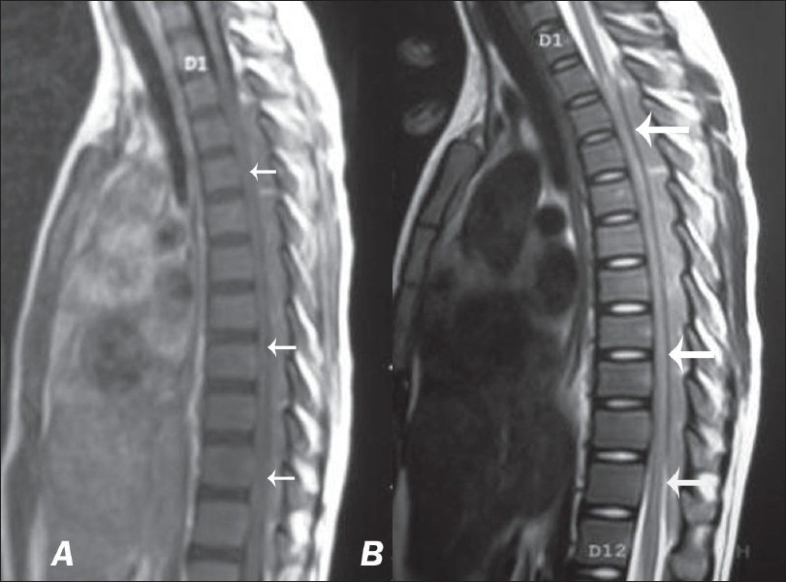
MRI of the dorsal spine showing an epidural mass that is hypointense in T1WI (figure 1A) and hyperintense in T2WI with a distinct line of dural demarcation (see arrows) in figure 1B
